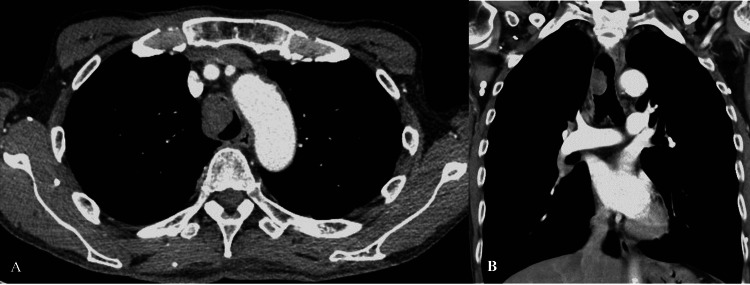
CT of the chest demonstrating a 2.4 × 2.7 cm lobulated mass abutting the right side of the lower trachea with nearly complete obstruction (A: axial view, B: coronal view).
